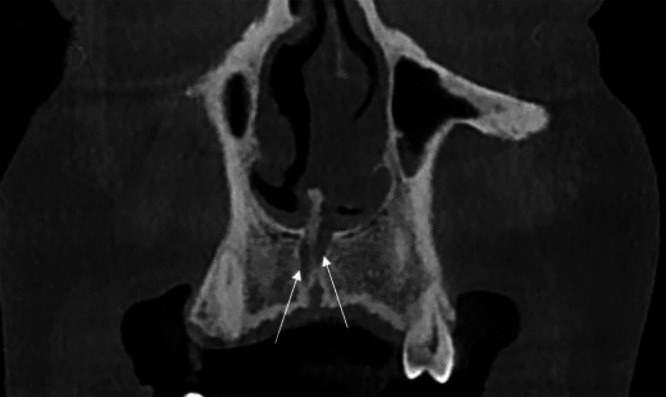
Coronal CBCT image of a 38-year-old male patient revealing a trifid nasopalatine canal, identified incidentally during pre-implant assessment. The image shows 2 of the 3 channels (arrows) separated by a bony septum, originating from the nasal floor and converging toward the incisive foramen, with the right and posterior canals aligned in this section.
